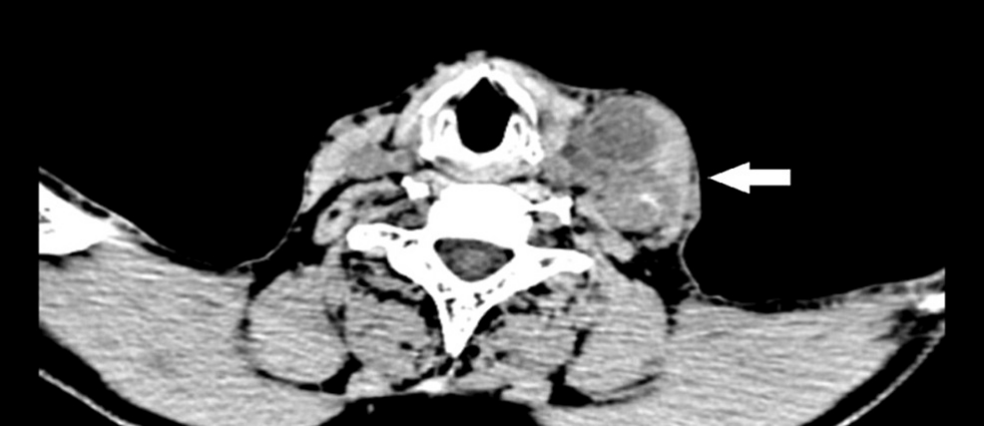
Computed tomography image of the neck (axial section) showing the extent of the lesion
